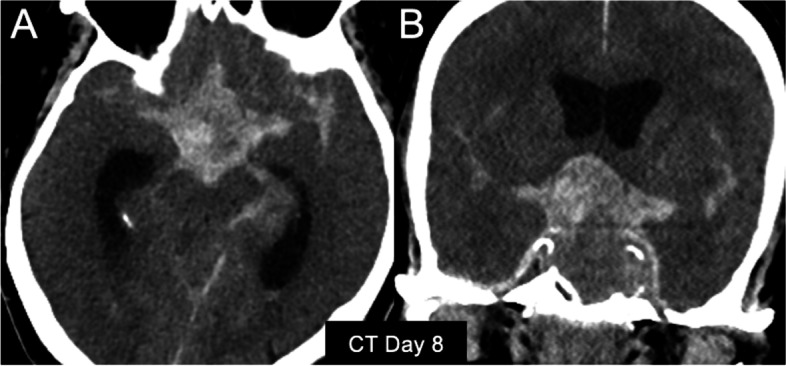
Unenhanced computed tomography images obtained immediately after the third attack of severe headache. A Axial and (B) coronal images showed massive subarachnoid haemorrhage distributed in the suprasellar cistern, bilateral sylvian fissures, perimesencephalic cistern, and ventricular system. Haemorrhage was present within the contour of the tumour. Hydrocephalus was noted
